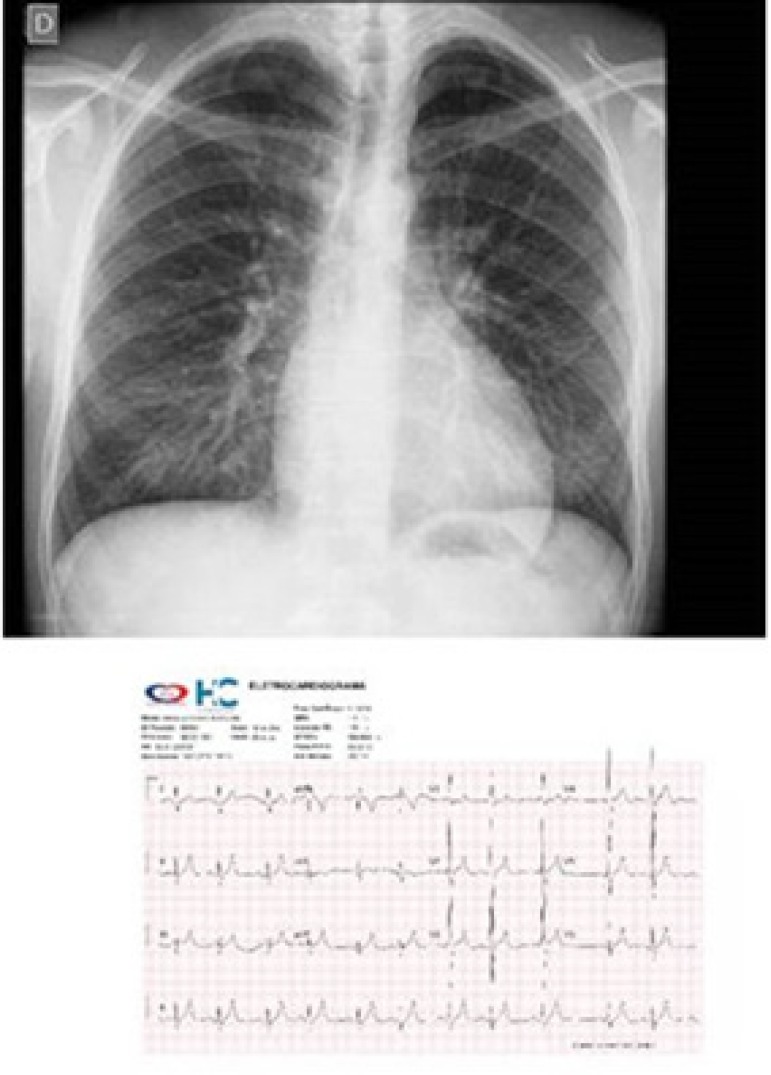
Chest X-ray showing the cardiac area within normal limits, with an
